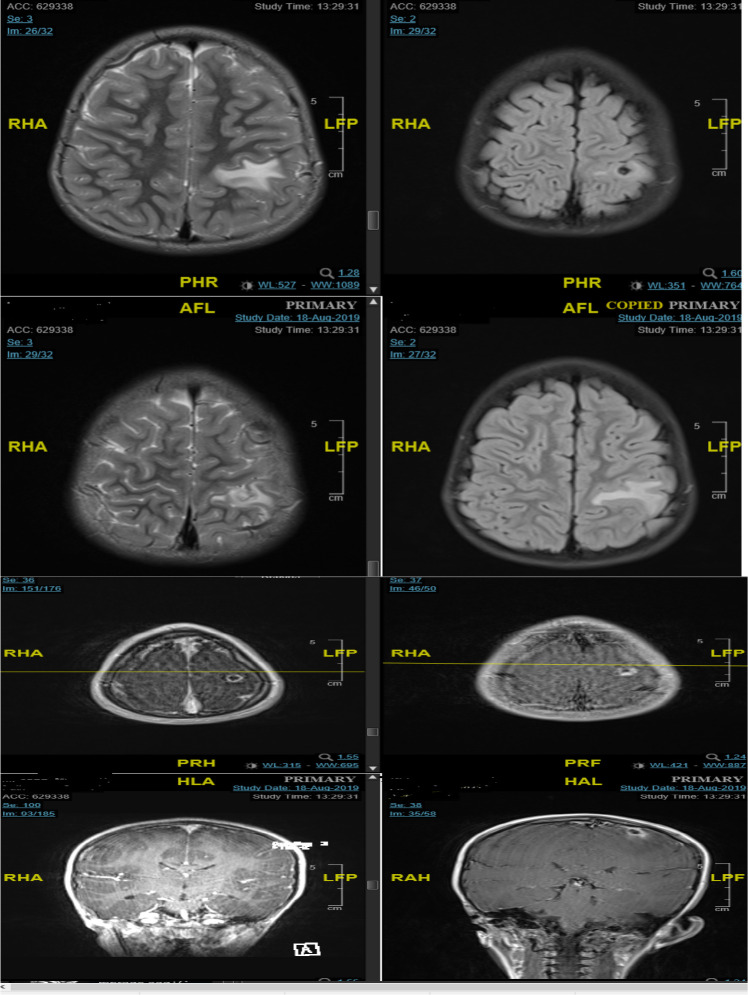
Left postcentral gyrus cortical lesion which measures 0.7 x 0.9 cm and display high T2, low T1 signal with no diffusion restriction. FLAIR images demonstrate low signal intensity with central foci of high signal. Furthermore, Perifocal edema seen involving post central gyrus. No other lesion identified. No evidence of blooming. Post contrast images demonstrate uniform wall ring enhancement.
